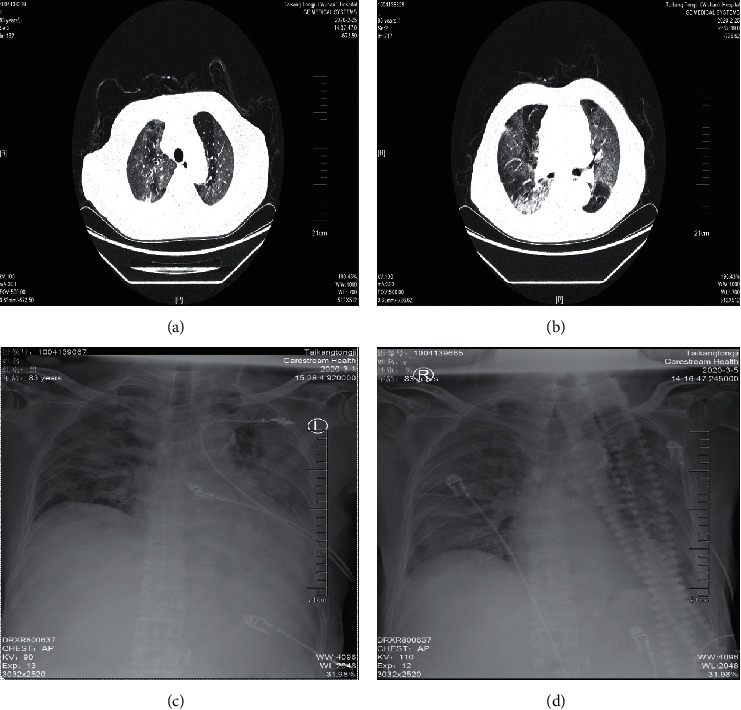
Imaging manifestation of chest CT and X-rays. (a) and (b) showed diffuse interlobular septum thickening in both lungs to form ground-glass opacities and thickening of the bronchial wall. (c) and (d) showed multipatchy shadows and diffused ground-like opacities in both lungs.
